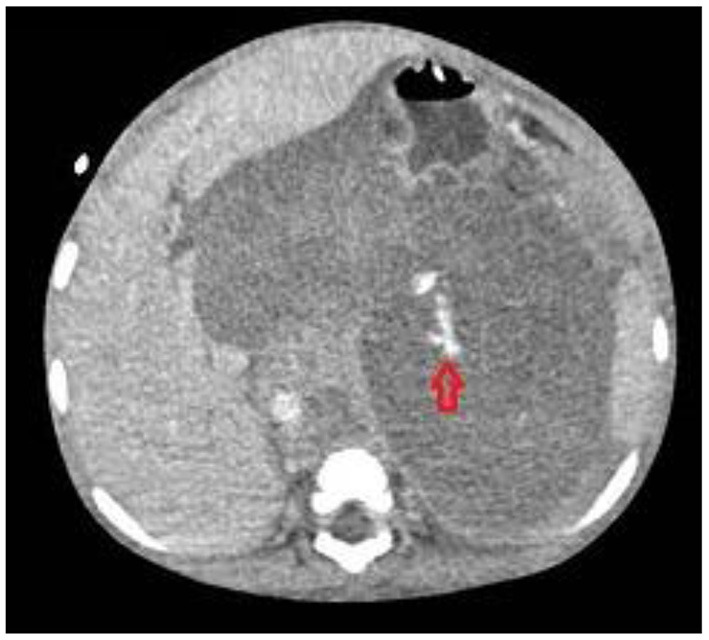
Bleeding from left diaphragmatic artery (CT scan: circle).
